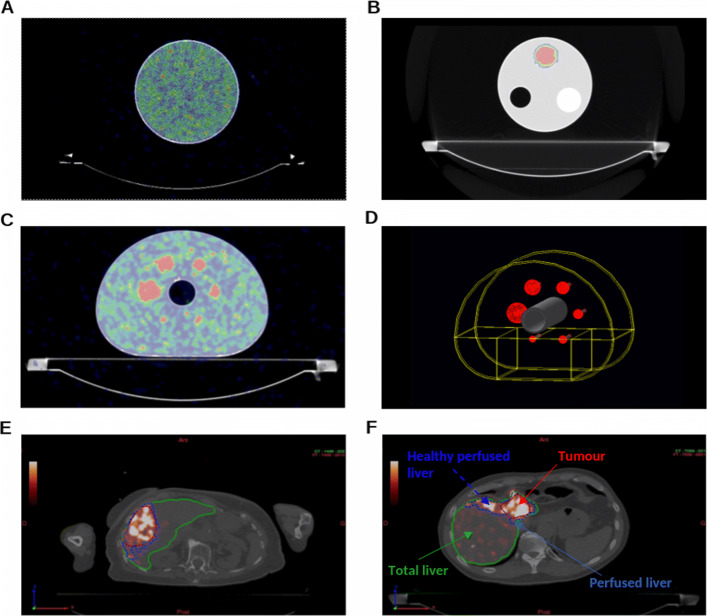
Axial slices of PET/CT images of A Ph1, B Ph2 and C Ph3. D Geometry of Ph3 modelled in GATE. E, F Examples of segmentation of liver VOIs for patients #2 and #3, respectively
